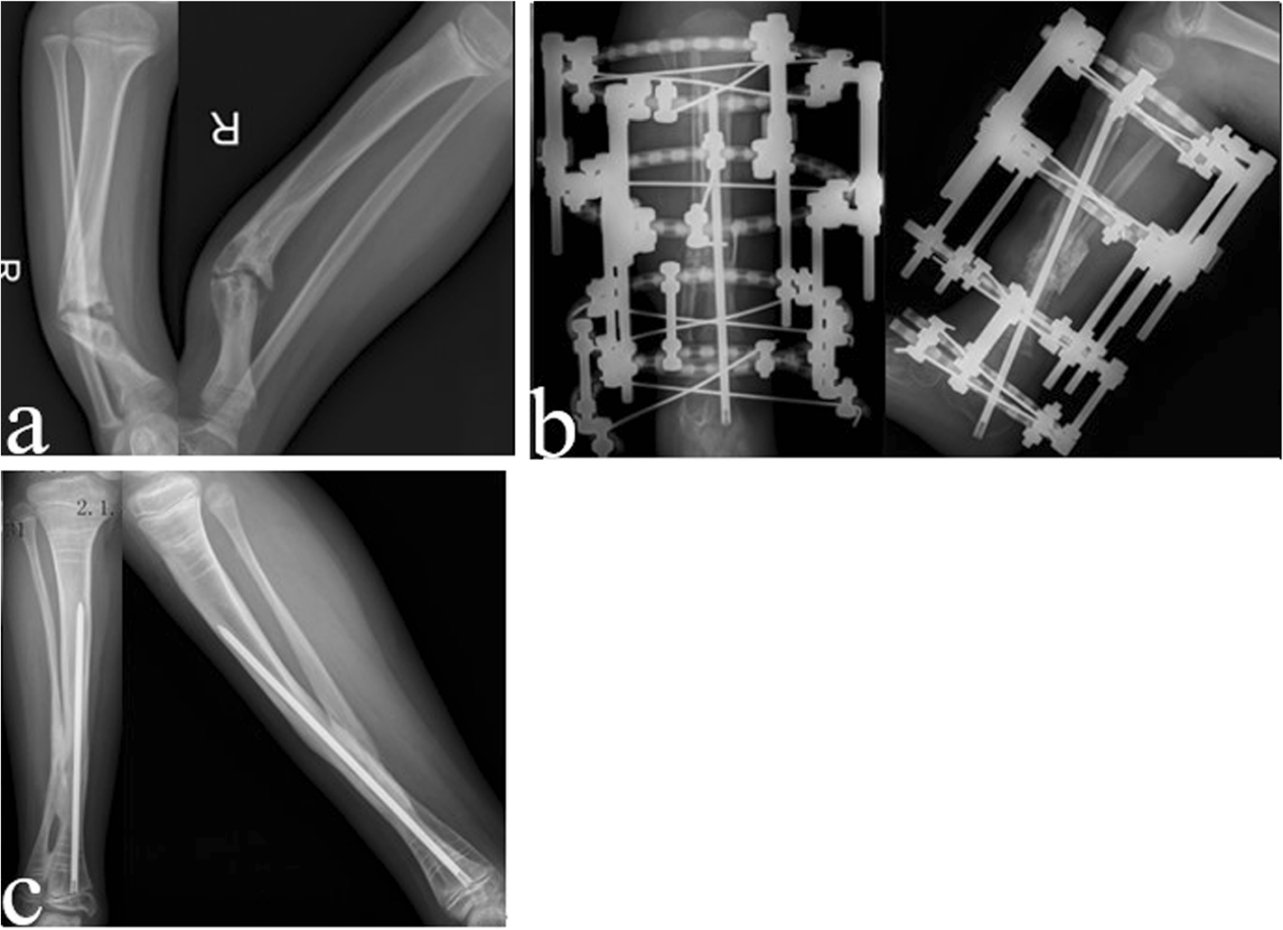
a Preoperative anteroposterior and lateral radiographs. b The X-ray radiograph, 4 days of post-operation. c Forty-eight months after the operation, X-ray shows that the tibia and fibula were well fused
